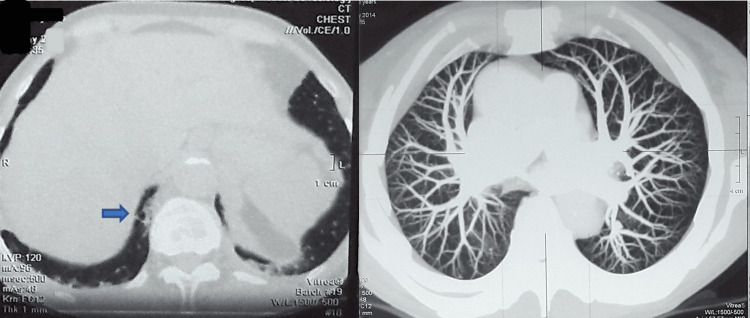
Chest multidetector computed tomography: bronchiectasis in the lower lobes (blue arrow). Bronchiectasis is most commonly a consequence of lower respiratory tract infections and can pose a risk factor for the occurrence of hemoptysis.
